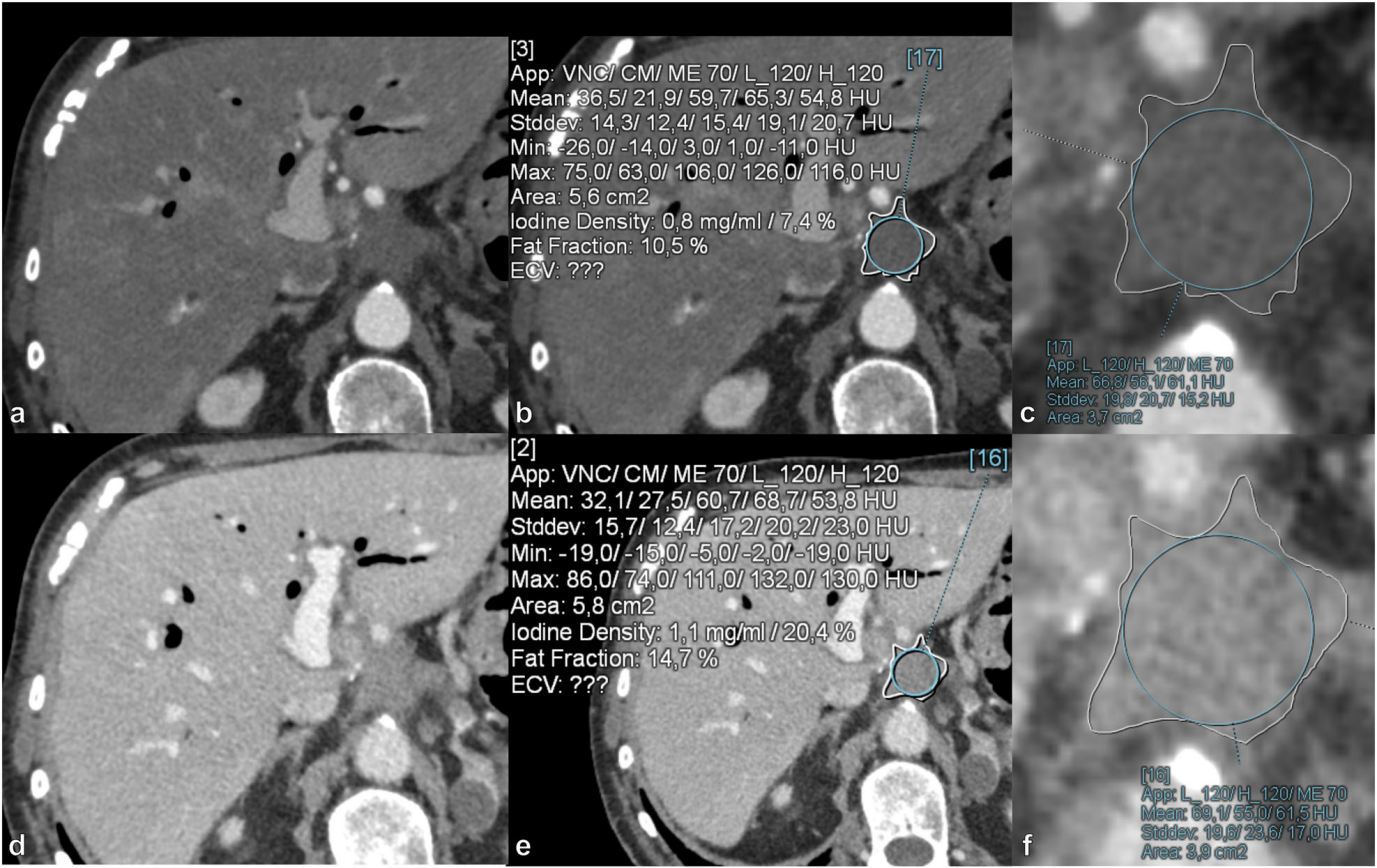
Axial contrast-enhanced abdominal PCD-CT scan in LAP in the first row (a, b, c), and in PVP in the second row (d, e, f). In the right column (c, f) are zoomed-in images of the ROIs. The images are of a 77-year-old male with LTR of PDAC post total pancreatectomy. Mean NIC in LAP was 0.08, and mean attenuation at 70 keV was 60.64 HU. According to the LAP model this yields a probability for LTR of 0.812 (81.2%). Mean IC in PVP was 1.03 mg/mL, and mean attenuation at 90 keV was 52.73 HU. According to the PVP model this yields a probability for LTR of 0.700 (70.0%). IC, Iodine concentration; LAP, Late arterial phase; LTR, Local tumor recurrence; NIC, Normalized iodine concentration; PCD-CT, Photon-counting detector computed tomography; PDAC, Pancreatic ductal adenocarcinoma; PVP, Portal venous phase; ROIs, Regions of interest
